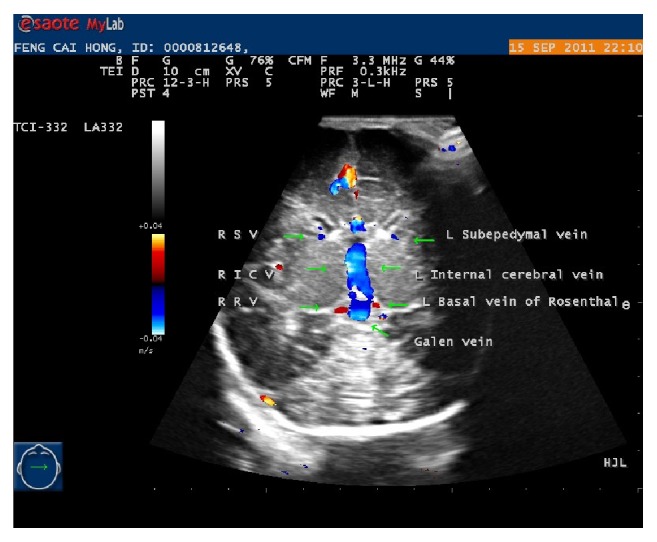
The color Doppler fontanel imaging of cranial venous system vessels at the coronal plane. The subependymal veins (SV), internal cerebral veins (ICV), Rosenthal veins (BV/RV), and Galen vein can be recognized from it.
